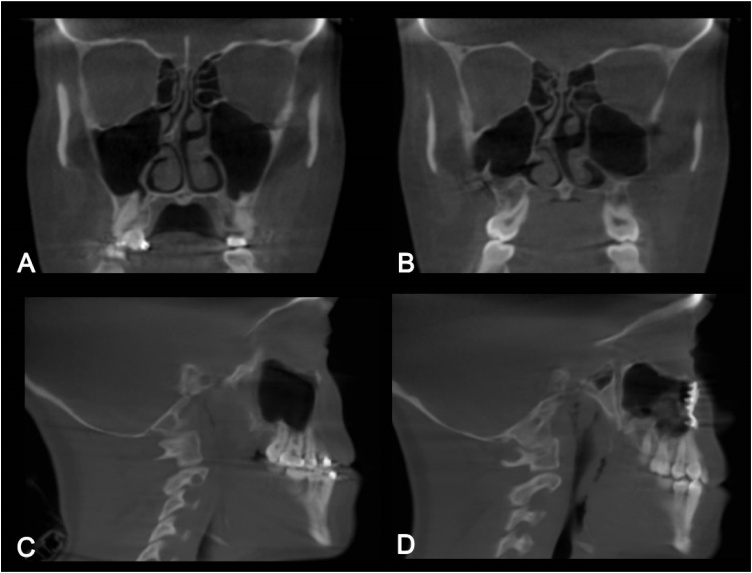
Anatomy of sinonasal region. (A) CT image (coronal section) of sinonasal region in preoperative period; (B) CT image (coronal section) of sinonasal region in postoperative period. (C) CT image (sagittal section) of sinus region in preoperative period; (D) CT image (sagittal section) of sinus region in postoperative period.
